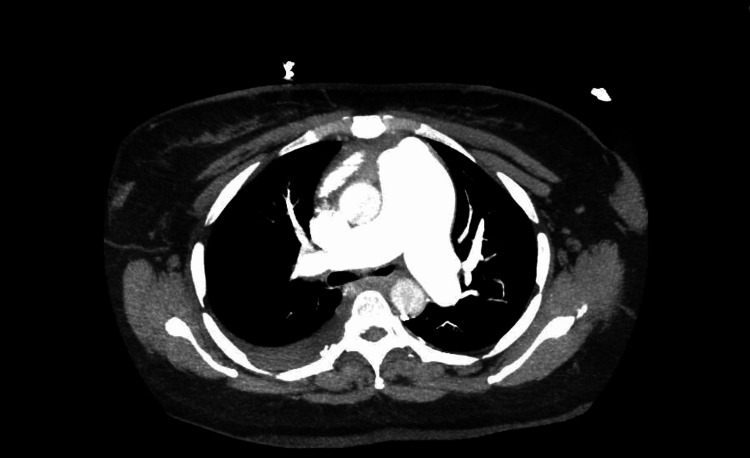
CTA of the chest showing no evidence of pulmonary embolism and enlarged diameter of pulmonary artery consistent with pulmonary hypertension. CTA: computed tomography angiography
